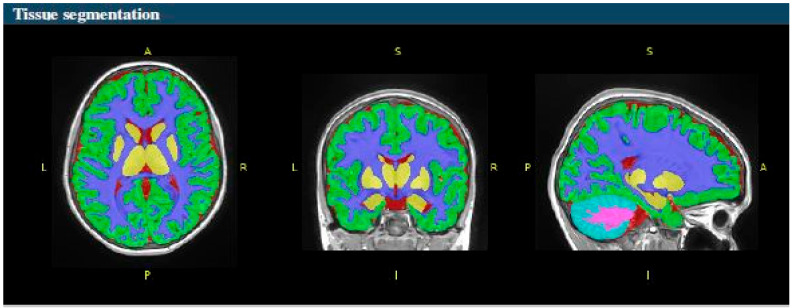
The 3D T1-weighted image tissue segmentation in axial (left image), coronal (middle image), and sagittal plane (right image); A—anterior; P—posterior; R—right; L—left; S—superior; I—inferior; Red: cerebrospinal fluid (CSF); Green: cortical gray matter (GM); Blue: white matter (WM); Yellow: basal ganglia (BG); Turquoise: cerebellum GM; Pink: cerebellum WM.
